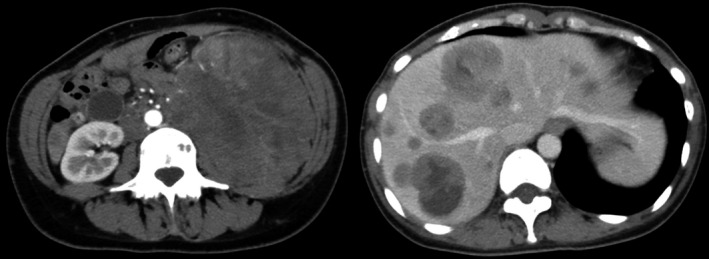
Computed tomography showed a solid tumor with irregular density in the left retroperitoneum and multiple liver metastases.
